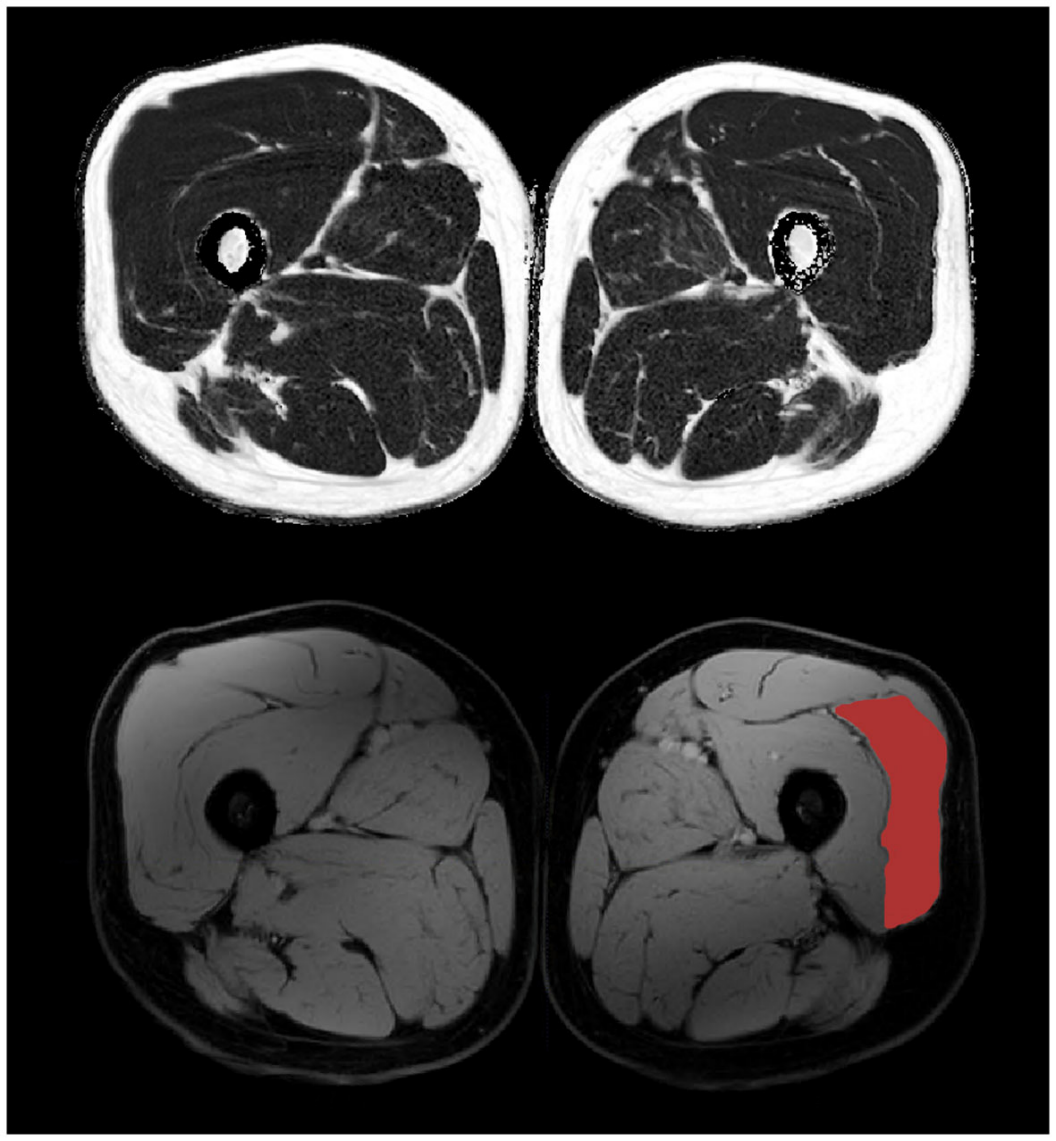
3-point Dixon mid-thigh MRI data with reconstructed fat (top) and water (bottom) maps. The vastus lateralis muscle is highlighted on the bottom right.
